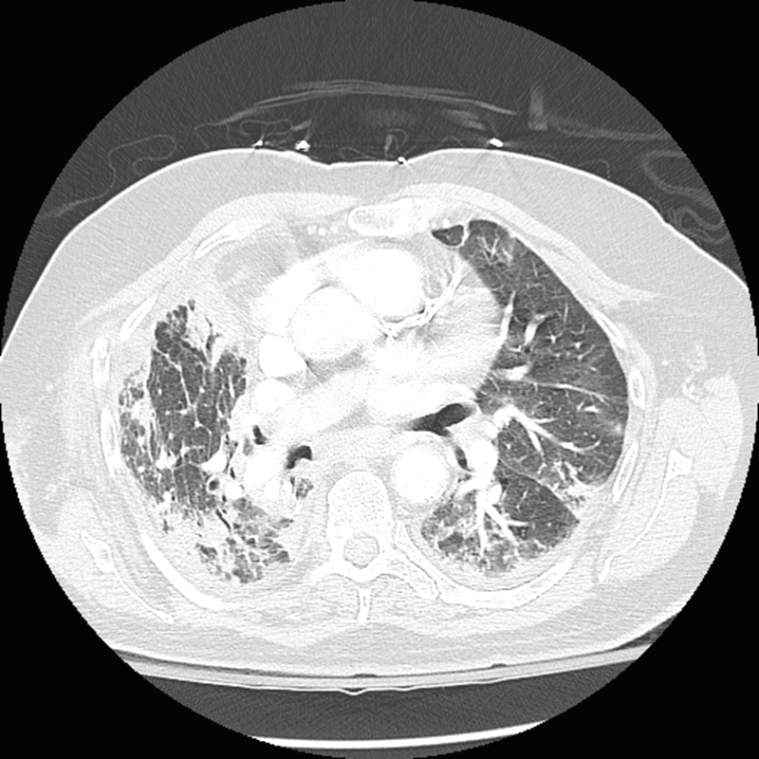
Computed tomography of the chest showing bilateral airspace opacities.
